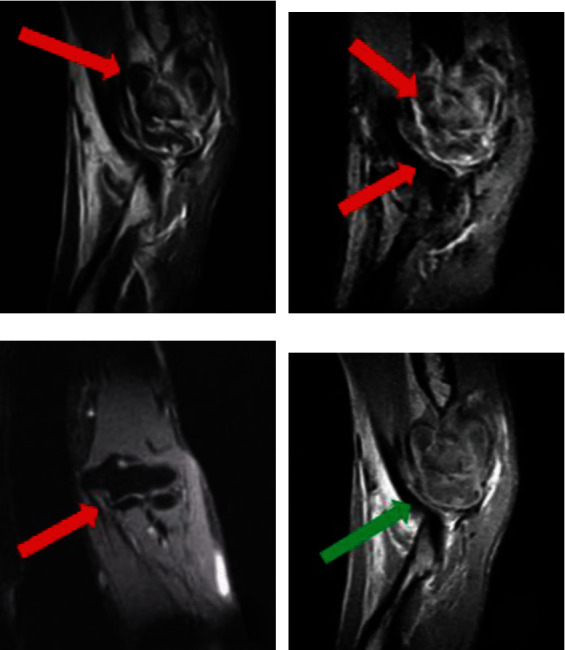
MR images before and after acupuncture treatment based on (a) MR image of elbow joint injury feature one before treatment, (b) MR image of elbow joint injury feature two before treatment, (c) MR image of elbow joint injury feature three before treatment, and (d) MR image after acupuncture treatment. The red arrow represents the injury site before treatment, and the green arrow represents the recovery position after treatment.
